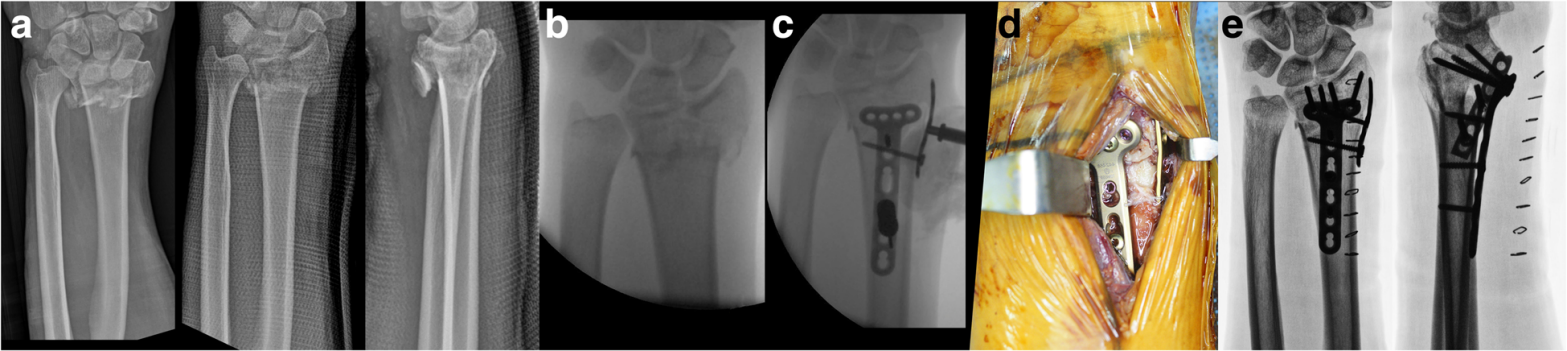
a Plain radiographs showing a metaphyseal comminuted fracture of the distal radius with radial length shortening. b Despite manipulations of the injured wrist under anesthesia, articular congruity was not restored because the radial styloid fragment was not reduced. c Use of the conventional flexor carpi radialis approach by splitting the PQ muscle from its origin to buttress the radial styloid fragment with the plate. d Orthogonal plate fixation of the distal radius through the conventional approach. e Postoperative radiographs showed the restoration of articular congruity and radial length
